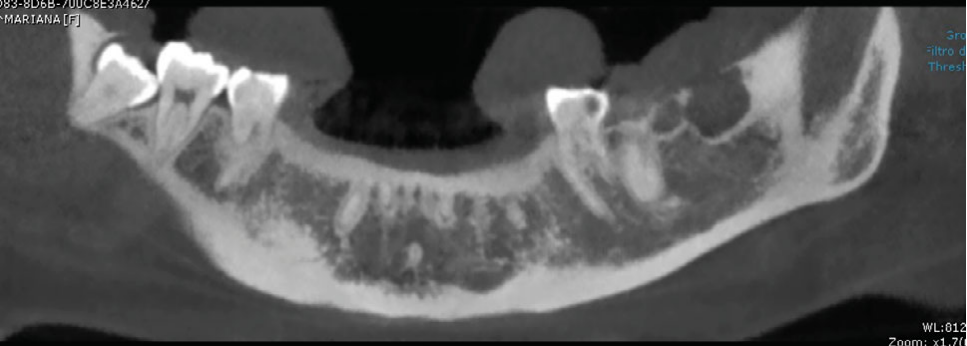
Cone beam with bone lysis is observed at the level of the retained third molar that extends toward the second molar and the posterior part of the mandibular body.
